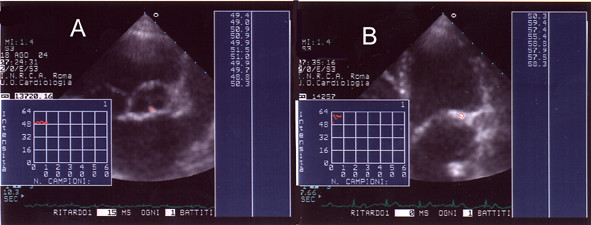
Score 5. Panel A: AD of aortic valve (parasternal scan: short axis); Panel B: AD of mitral valve (apical scan).
